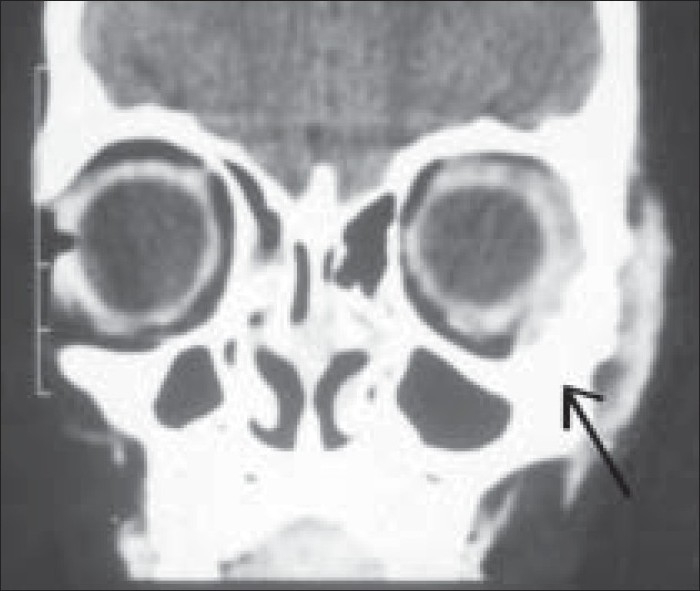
Patient 1 – CT scan of the orbit showing an infiltrative mass with irregular margins arising from the lateral orbital wall
